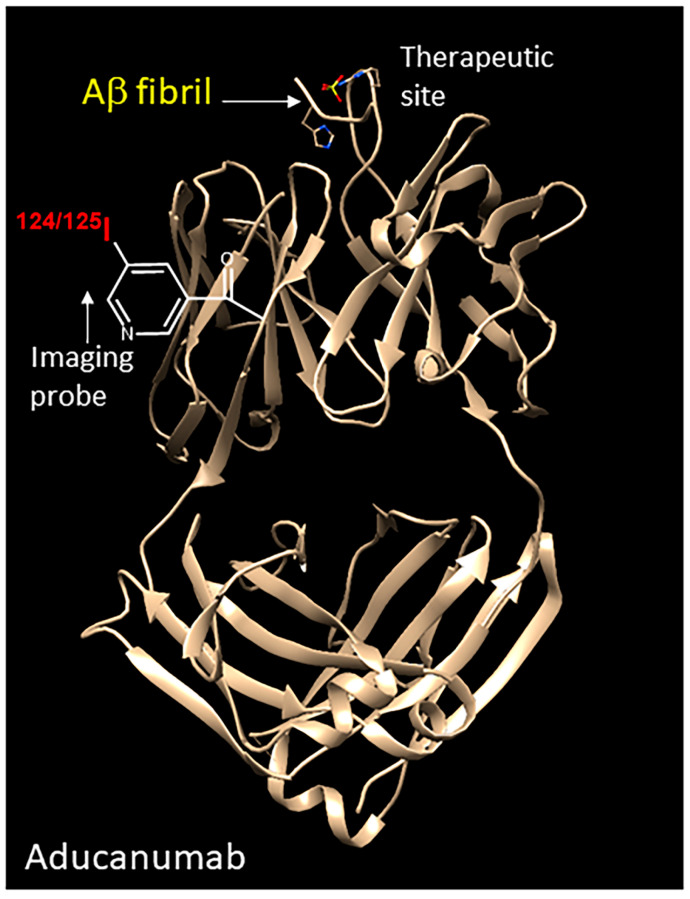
Aducanumab Aβ complex (PDB 6CO3 [32]). Structure of Aducanumab with the Ab fibril (yellow text) bound at the top. Similar to our results with [^125^I]IPC-Lecanemab, both Aducanumab and Lecanemab may be radiolabeled with iodine-124 (red text) to produce [^124^I]IPC-Aducanumab and [^124^I]IPC-Lecanemab, which can be used for PET imaging. Along with a focused ultrasound intervention, delivery of these therapeutic antibodies can be measured.
